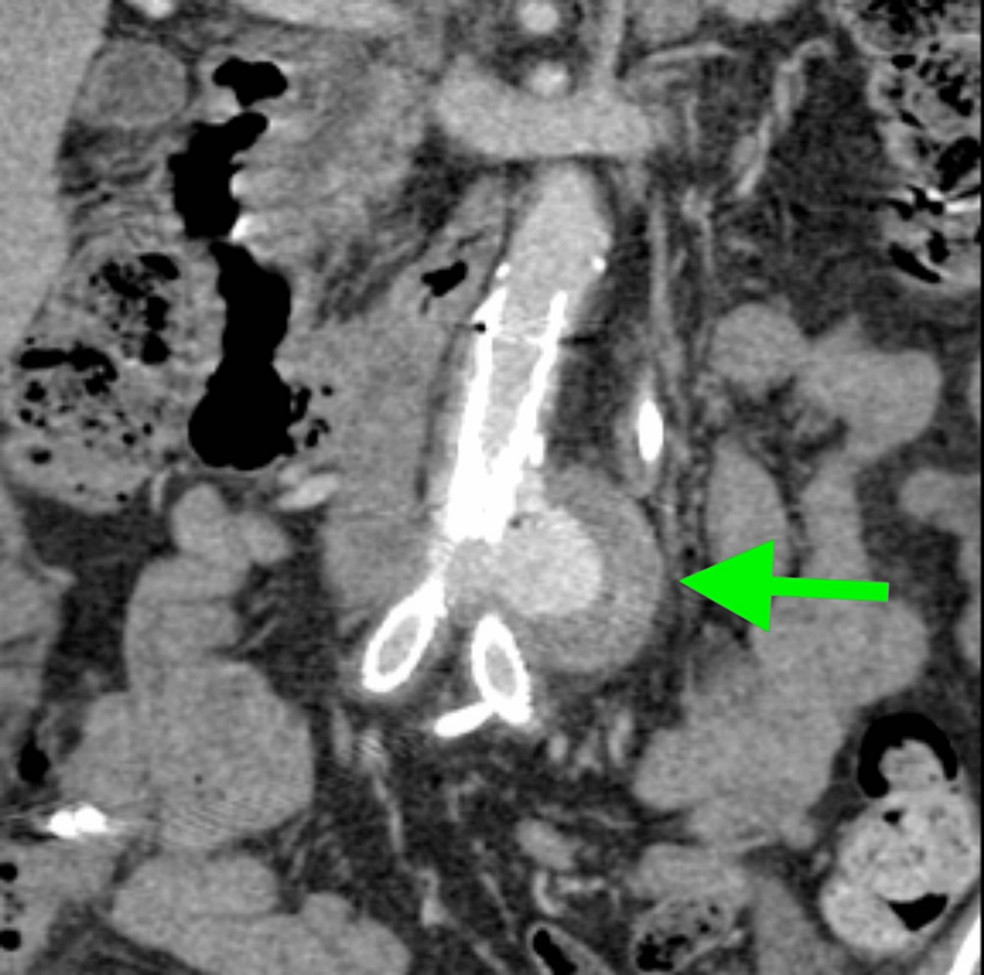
Whole body CTA on admission showing endoleak formation (green arrow). CTA: computed tomography angiography
